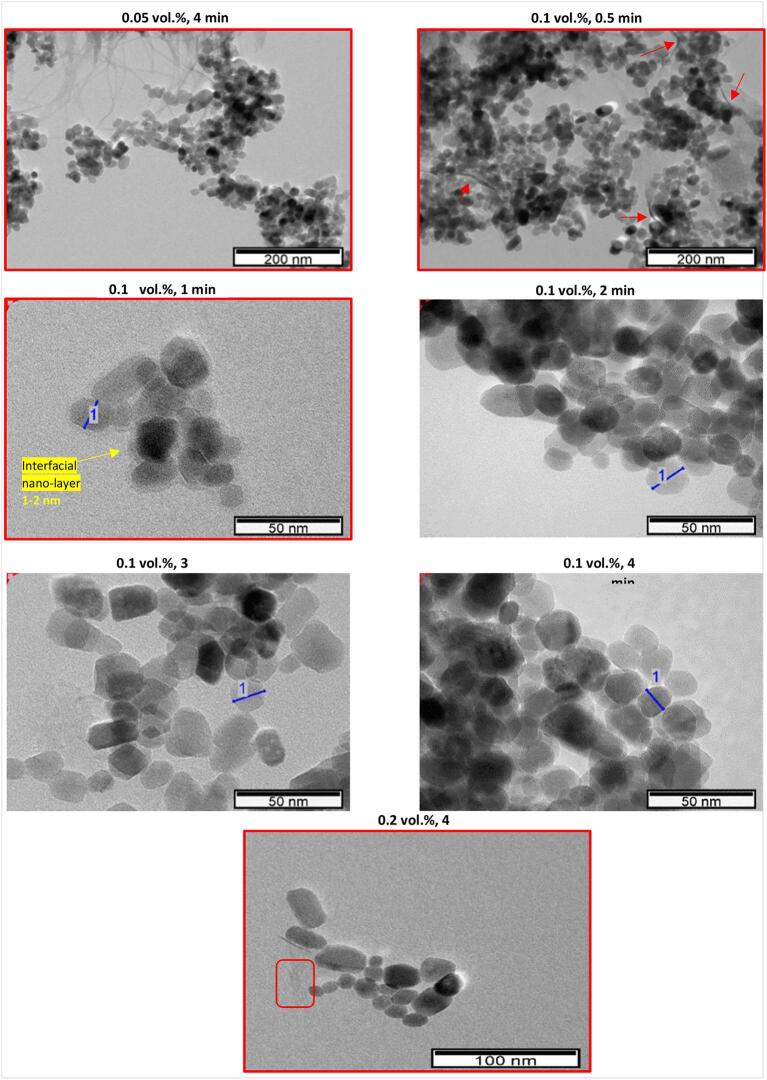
TEM images of ZnO-DW nanofluids at different concentrations and ultrasonicated at 100% amplitude.
